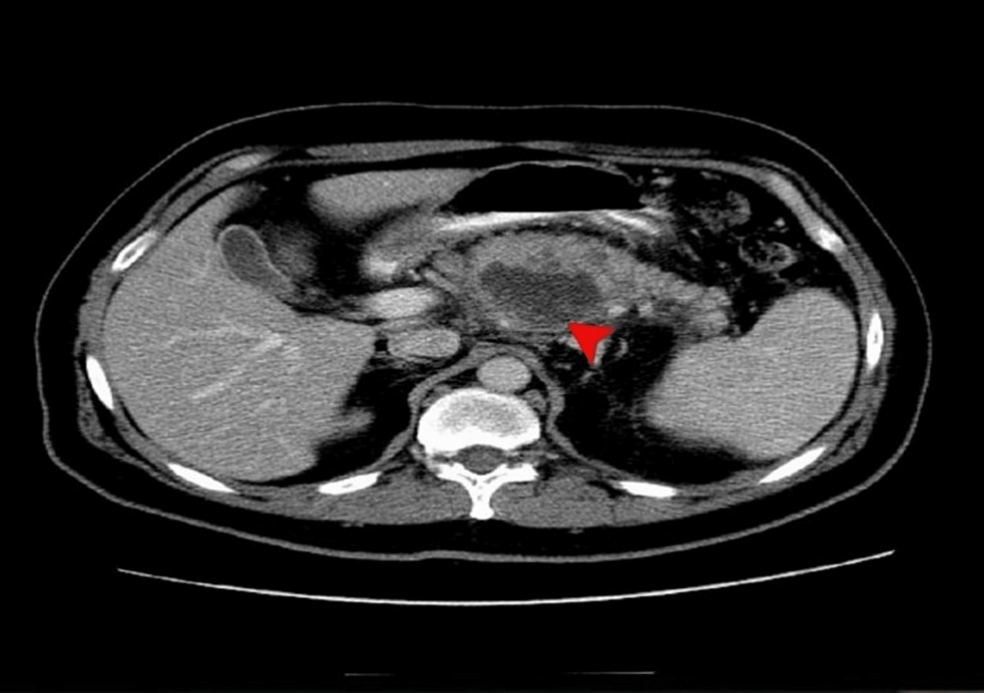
CT abdomen and pelvis with contrast (axial view) The red arrow represents a mixed density heterogeneously enhancing mass with large areas of necrosis in the body of the pancreas with encasement of the splenic artery and vein likely representing neoplastic pancreatic body mass - mucinous cystadenocarcinoma.
